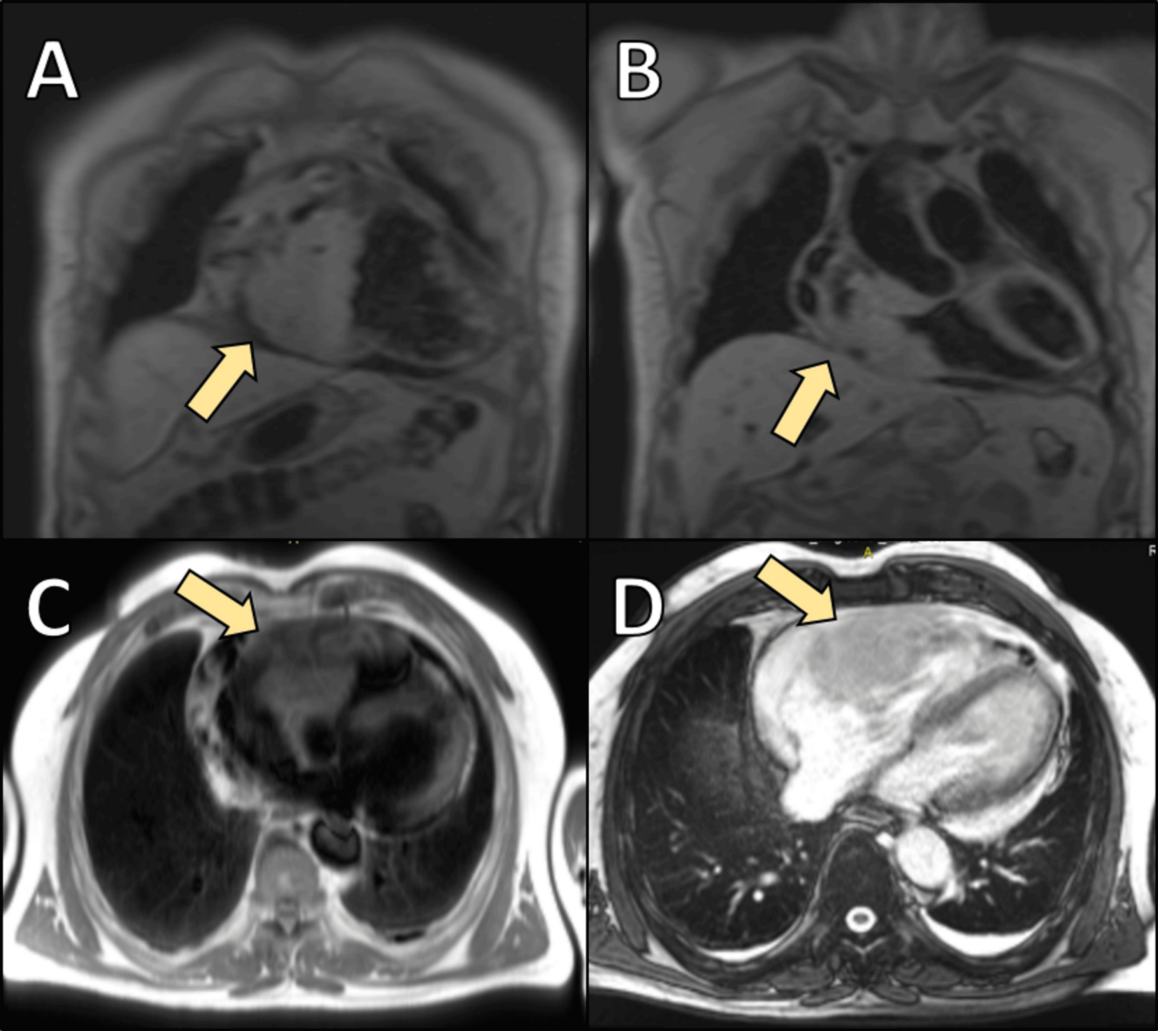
Magnetic resonance imaging (MRI) of a cardiac tumor in the right atrium and ventricle. (A) Coronal reconstruction of a T1-weighted sequence showing the distribution of the mass within the right atrium (arrows). (B) Coronal plane of a T1-weighted sequence highlighting the tumor's extension into the right ventricle. (C) Transverse plane of a T1-weighted sequence depicting the mass within the right ventricle. (D) Transverse cross-sectional plane of a T2-weighted sequence demonstrating the mass's extension and spatial orientation.
